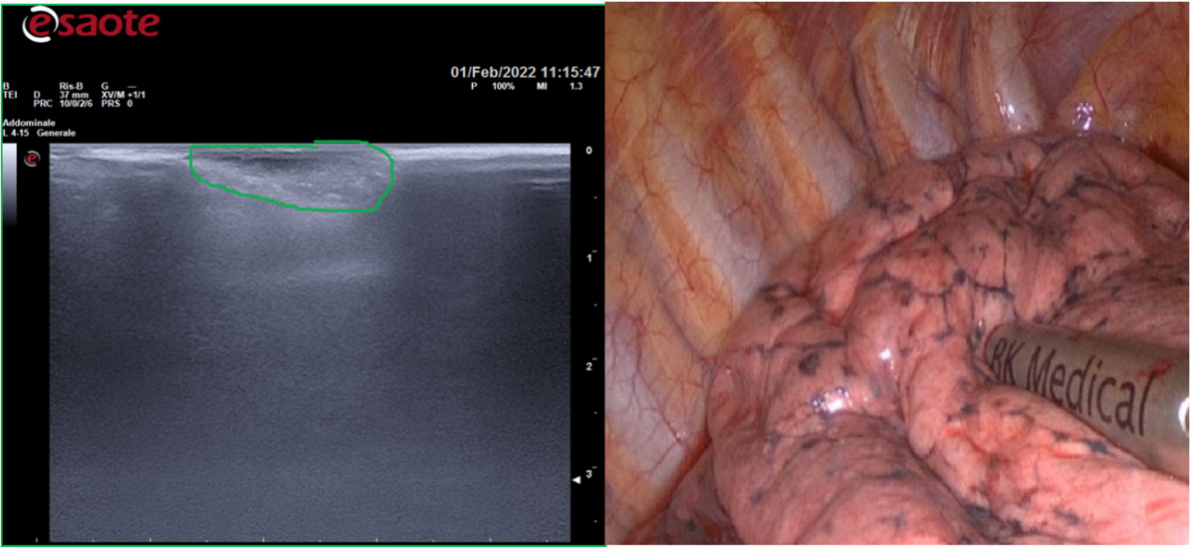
The probe was placed in the region of the target lesion. The ultrasound consents to distinguish the ground-glass opacity from the lung parenchyma.
